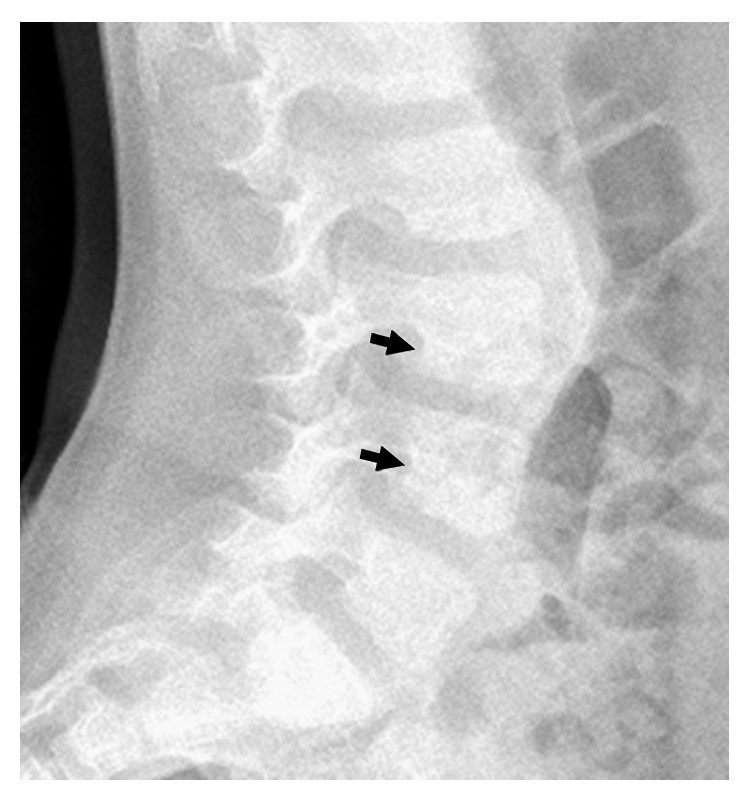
Lateral spine radiograph showed exaggerated lumbar lordosis associated with scalloping of the posterior end-plates (arrows).
